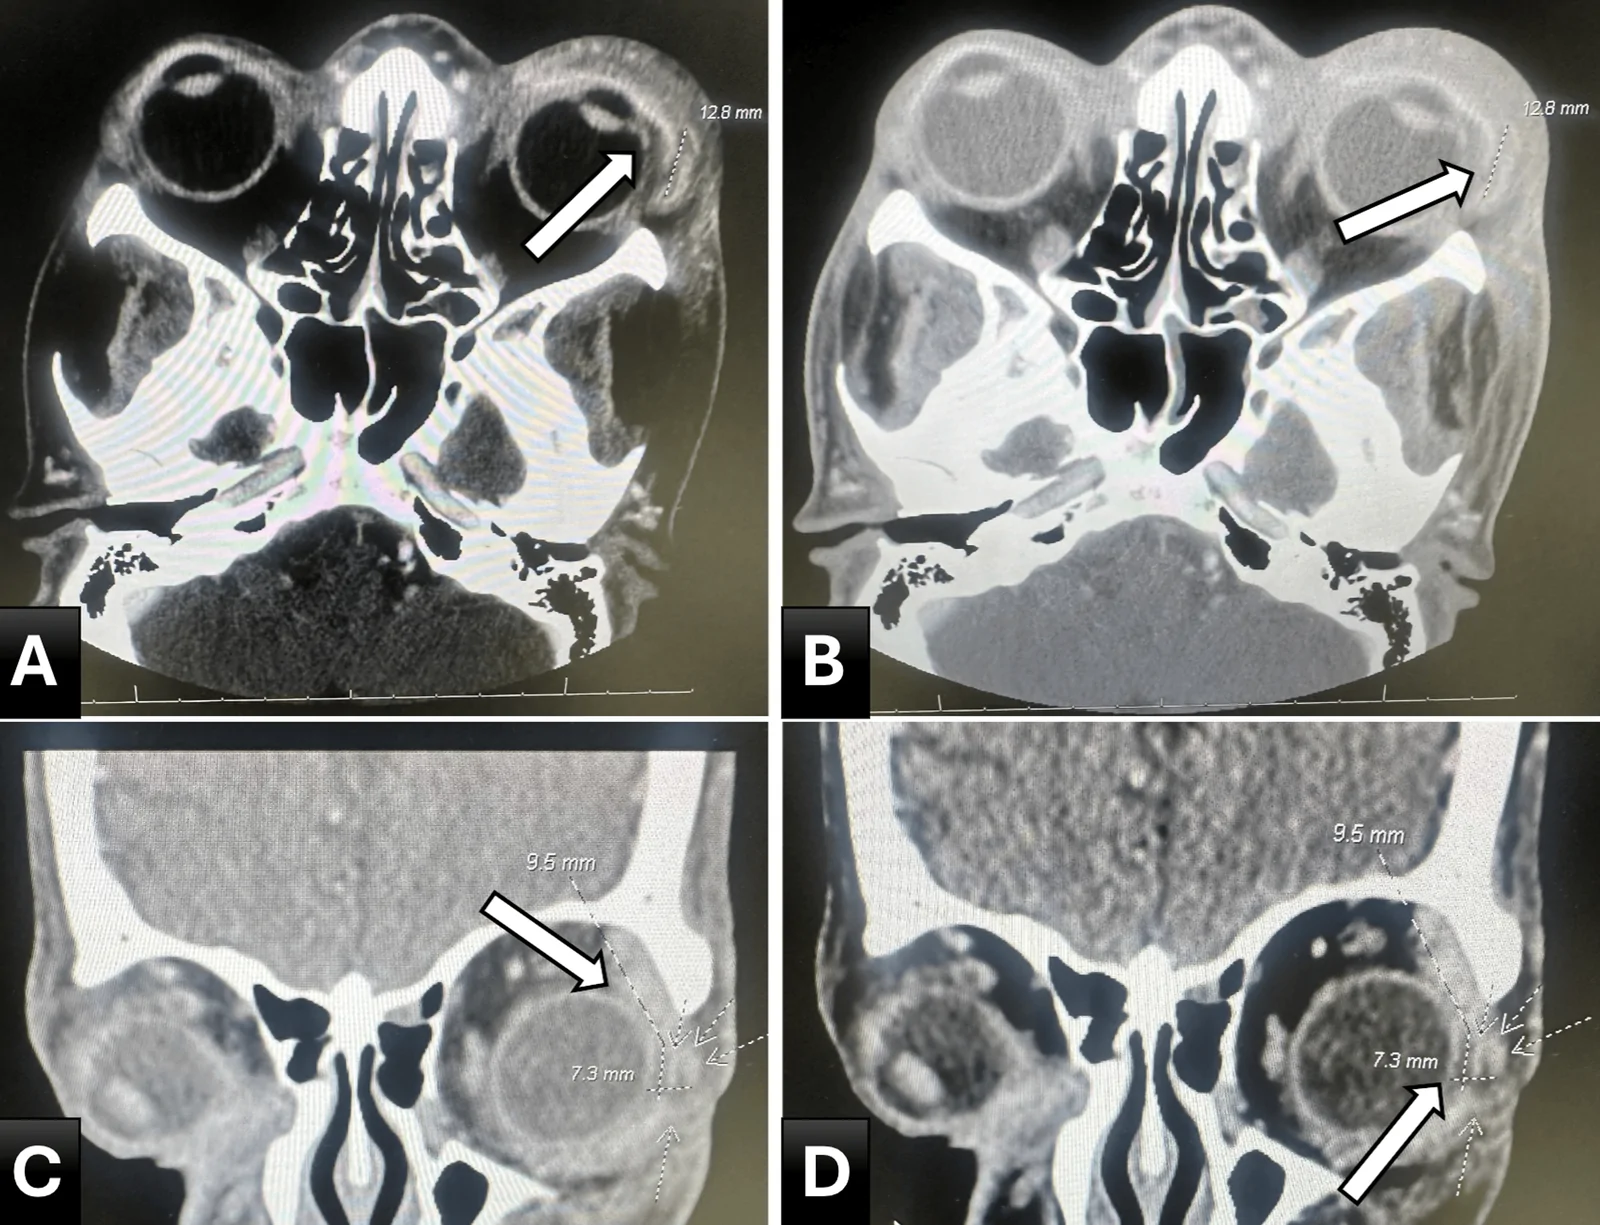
Serial computed tomography imaging of the left orbit demonstrating progressive superior orbital soft tissue thickening (A, B) Initial contrast-enhanced CT of the orbits demonstrates diffuse preseptal and lateral periorbital soft tissue thickening centered within the superolateral left orbit (white arrows), with associated enlargement of the left lacrimal gland and a focal peripherally enhancing hypodense component concerning for evolving phlegmon or abscess. The intraconal fat, extraocular muscles, optic nerve sheath complexes, and orbital osseous structures are preserved. (C, D) Follow-up CT performed after prolonged intravenous antimicrobial therapy demonstrates persistent and mildly progressive soft tissue thickening centered along the temporal aspect of the left orbit (white arrows), with persistent reactive lacrimal gland enlargement and extension into the infraorbital soft tissues despite treatment. The persistence of enhancing orbital soft tissue without a drainable abscess prompted tissue biopsy, which ultimately established invasive conjunctival squamous cell carcinoma.
